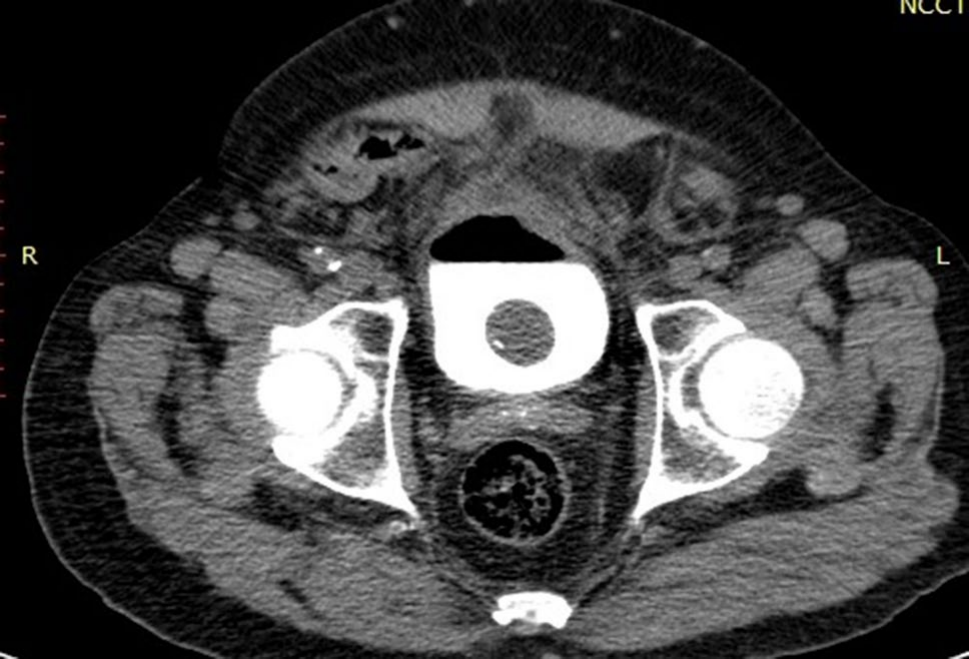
In the same patient, CT cystogram axial view with post indwelling urethral catheter for 10 days after the initial presentation showing the contrast in the UB with resolving of the extravasation
